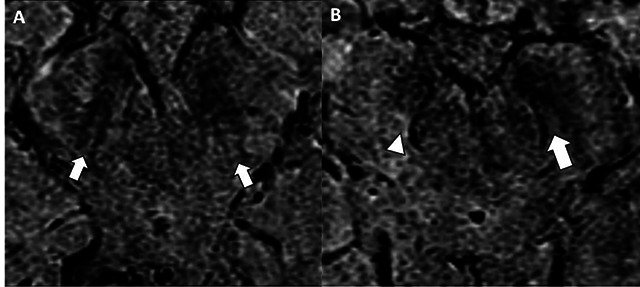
Nigrosome-1. Assessment of the substantia nigra using 3T high resolution susceptibility-weighted MR imaging at the level of nigrosome-1 in 2 different patients. The control subject (A) shows normal nigrosome-1 present bilaterally (arrow) and the PD patient (B) demonstrates right nigrosome-1 absent (arrowhead).
